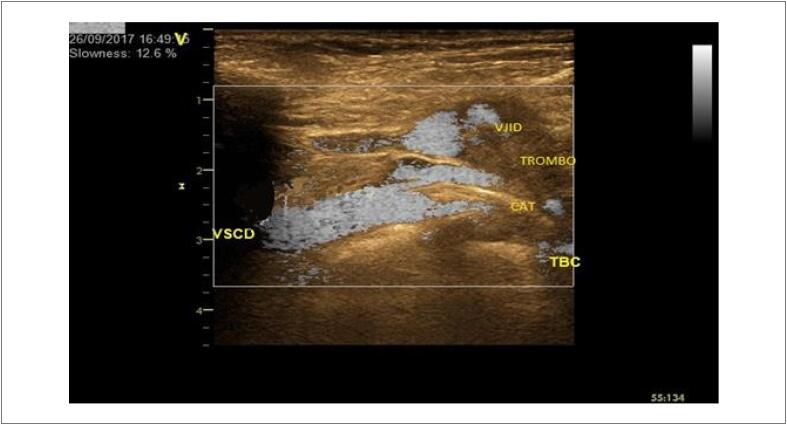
Ultrassonografia vascular (USV) na região supraclavicular proximal, evidenciando trombose na veia jugular interna direita (VJID) proximal, tronco venoso braquiocefálico (TBC) e veia subclávia direita proximal (VSCD). Observa-se o cateter central em VSCD direcionado ao TBC.
